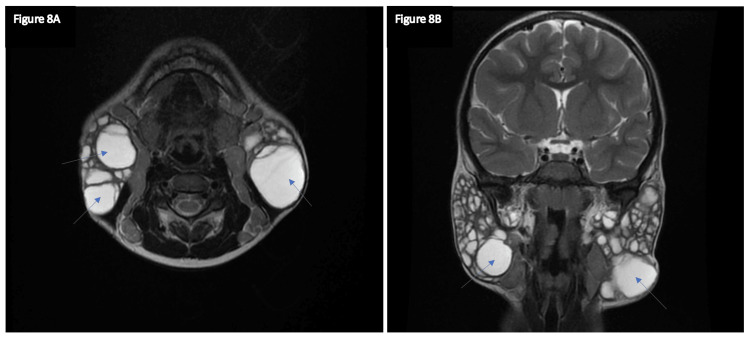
MRI Images in Axial view (A) and Coronal view (B) showing diffuse enlargement of parotid glands (blue arrows) suggestive of benign lymphoepithelial lesions
